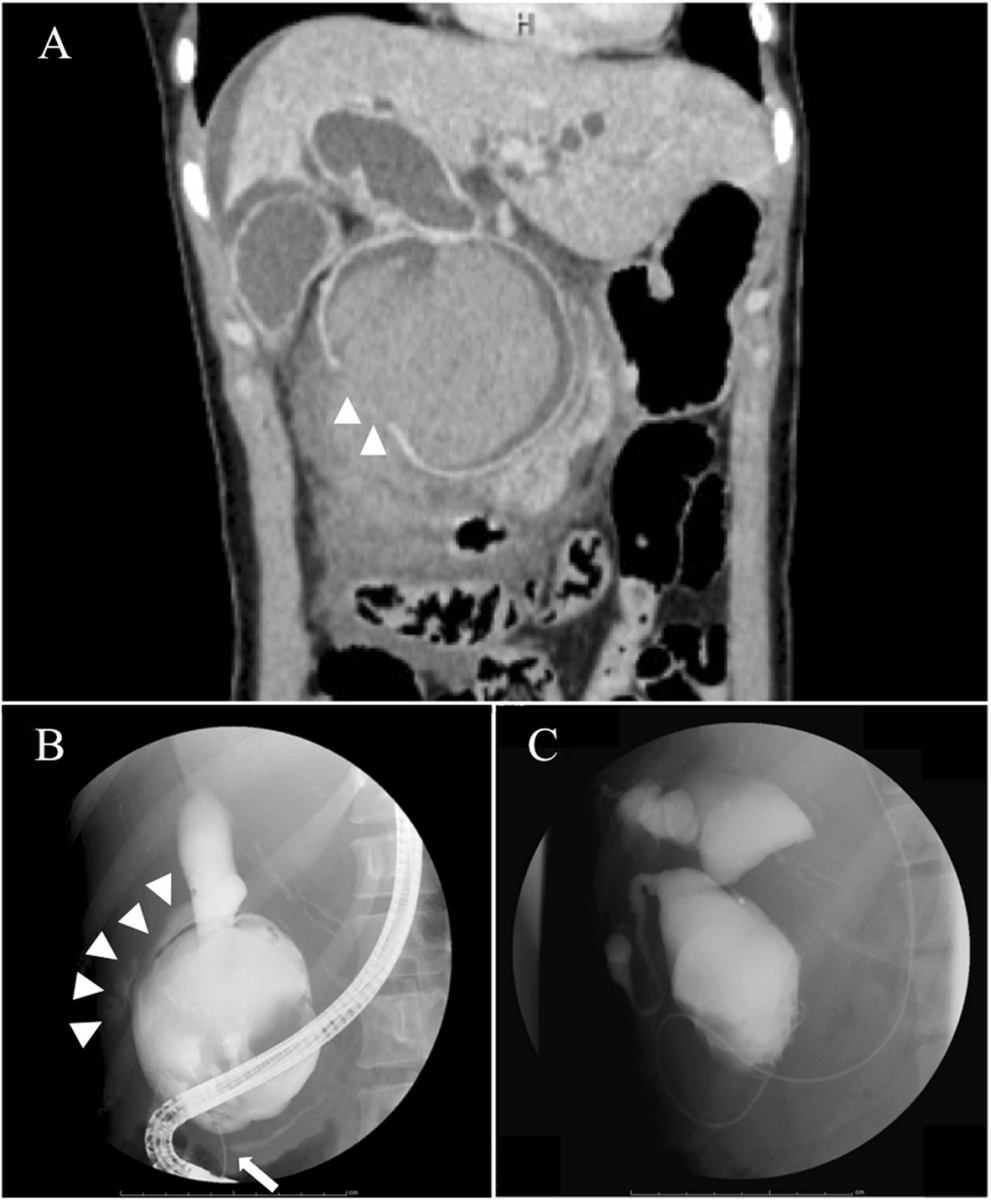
Preoperative imaging findings. a Urgent contrast-enhanced abdominal computed tomography scan, showing a huge cyst with hemorrhaging in the common bile duct (CBD) and a defect of the contrast medium at the right-side wall of the CBD (*arrowheads*). Fluid collection around the CBD, mainly in the retroperitoneal space, also is observed. b Endoscopic retrograde cholangiopancreatogram, showing leakage of the contrast medium from the right-side wall of the dilated CBD (*arrowheads*) with anomalous pancreaticobiliary ductal union (*arrows*). c Cholangiogram taken by the nasal biliary drainage tube, showing dilation of both intra- and extrahepatic bile ducts, indicating a type IVa choledochal cyst according to Todani’s classification
